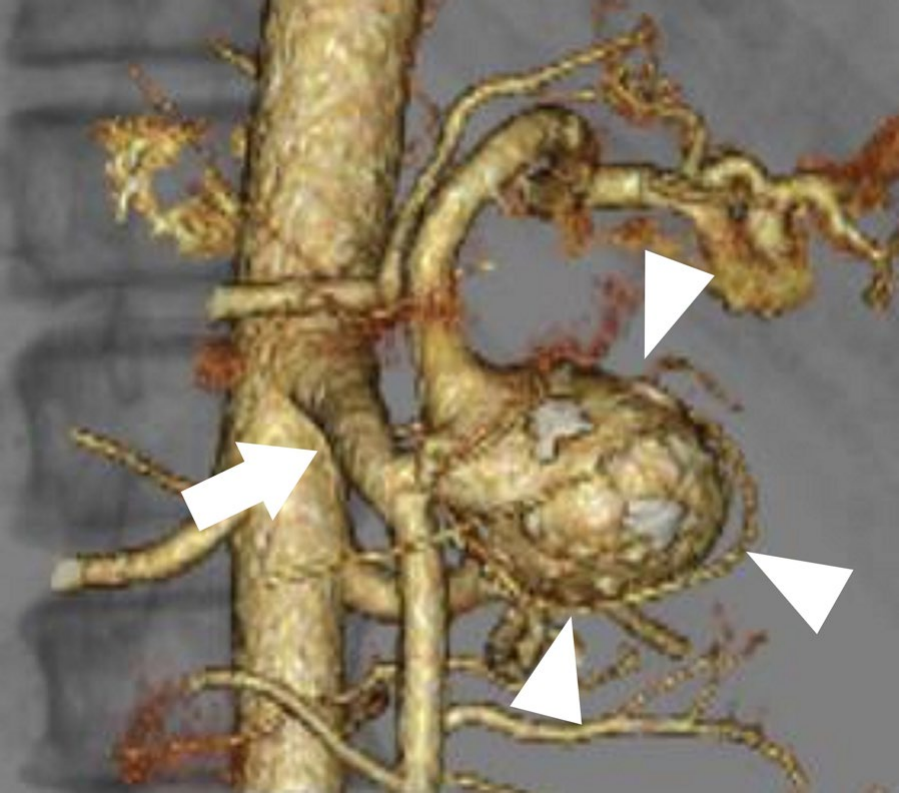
Preoperative computed tomographic angiography findings. The splenic artery bifurcated from the superior mesenteric artery (white arrow), and a 38-mm splenic artery aneurysm (white triangle) was visible immediately after the bifurcation
